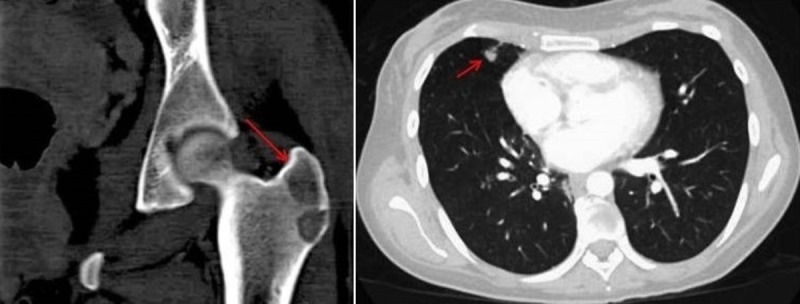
Radiographic evidence of progression at one year from the start of erlotinib treatment Left panel is the representative sagittal section image of computed tomography (CT) without contrast of the left hip showing a new lytic lesion at the base of the left greater trochanter measuring 1.4 cm x 1.3 cm. Right panel is the axial section of CT with intravenous contrast of the chest depicting new lung nodules in the right lower lobe consistent with progressive disease.
